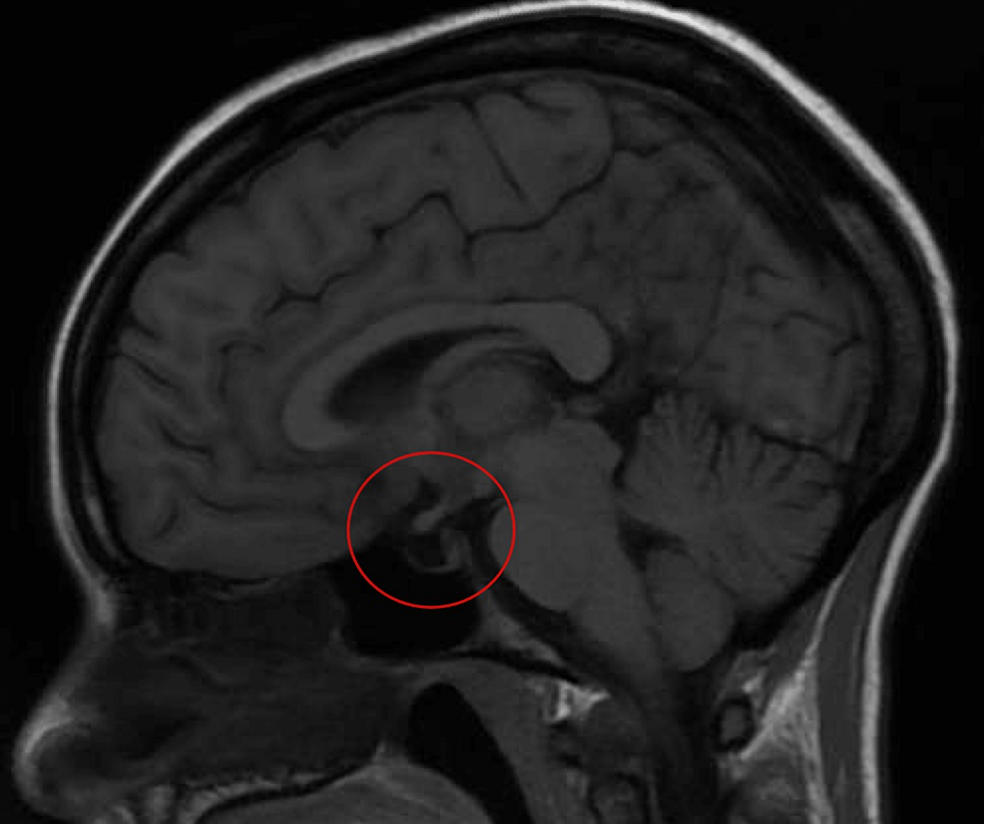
Magnetic resonance imaging (MRI) of the head for this patient confirming the absence of anatomical structures within the sella turcica
